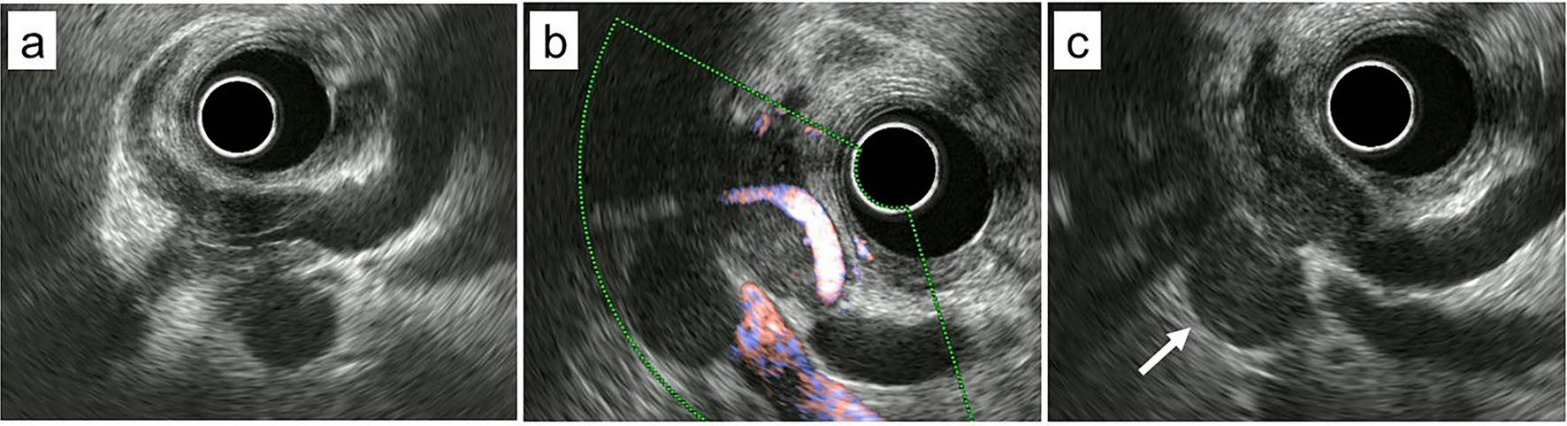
Endoscopic ultrasonography. a The thickened bile duct shows a smooth inner border echo. b The lumen of proper hepatic artery penetrating the thickened wall is preserved. c A 2-cm mass (arrow) is found close to the lesion, which is considered an enlarged lymph node. The boundary between the bile duct and the mass is clear and no continuity is observed
